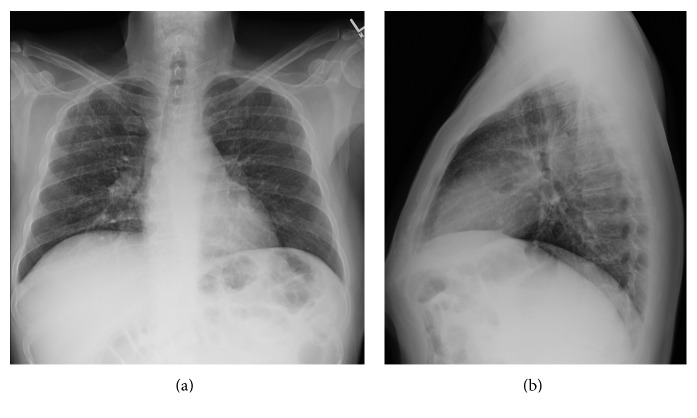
Chest roentgenogram four months after hospitalization. (a) PA and (b) lateral chest radiographs post treatment four months later demonstrated marked improvements.
